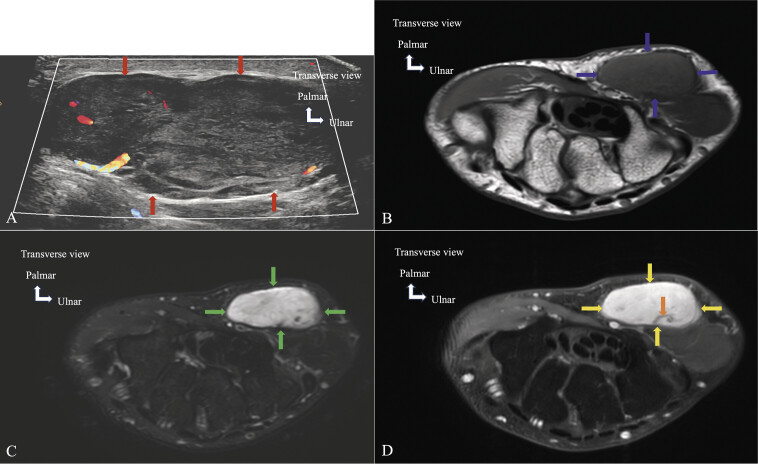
Angioleiomyoma. Transverse ultrasound image
